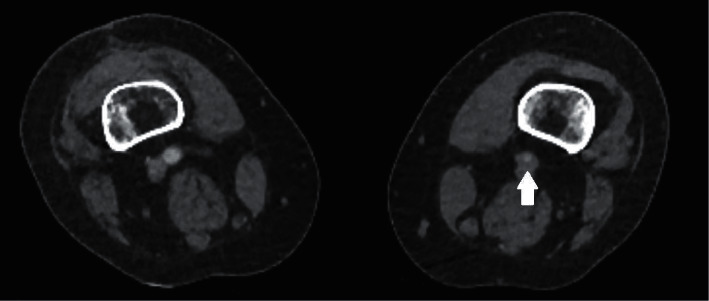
A CT angiogram demonstrates an occlusive thrombus (white arrow) of the left common femoral artery.
